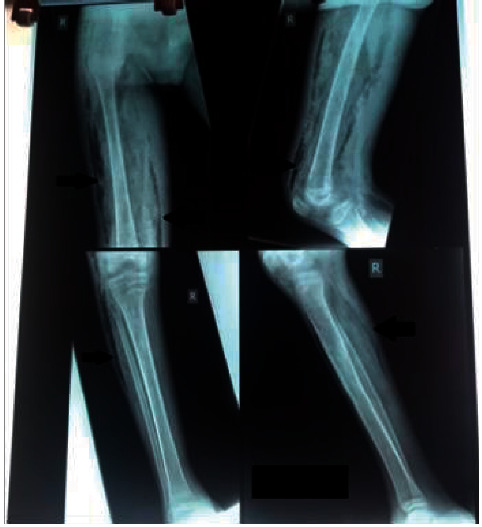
X-ray images showing gas pockets in the right thigh and leg.
